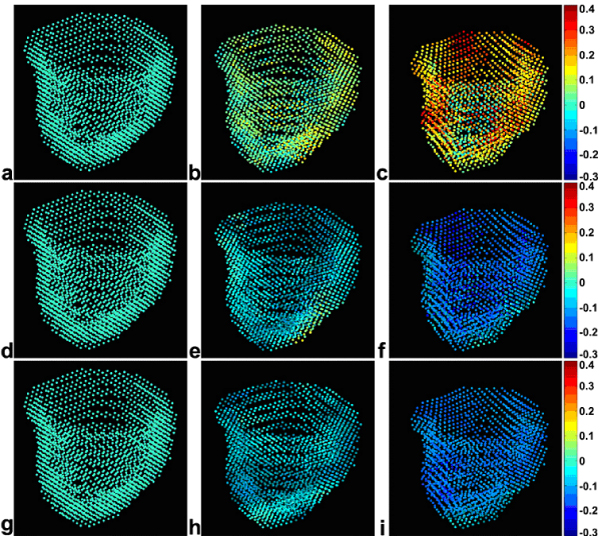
Radial (a-c), circumferential (d-f), and longitudinal (g-i) strains computed throughout the LV from 3D cine DENSE MRI of a normal volunteer. Data are shown at end diastole (a, d, g), mid systole (b, e, h), and end systole (c, f, i). Three of 22 cardiac phases are displayed. Individual dots represent displacement, while color represents strain.
